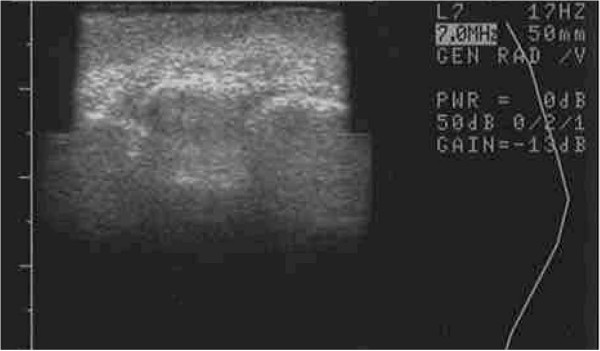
Ultrasound image of left hand (DEP-SVF treated) coronal view of thenar web space of a 69-year-old female 3 months post lipotransfer.
